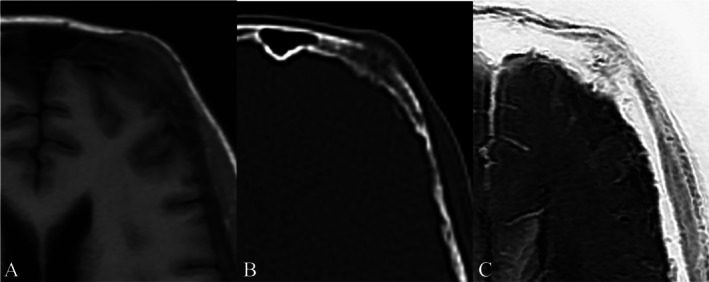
Axial non‐contrast T1‐weighted MRI (A) demonstrates a metastatic soft‐tissue lesion involving the left frontal bone in a patient with lung adenocarcinoma. Corresponding axial CT in bone window (B) and grayscale‐inverted susceptibility‐weighted imaging (SWI) (C) confirm associated osteolytic changes.
